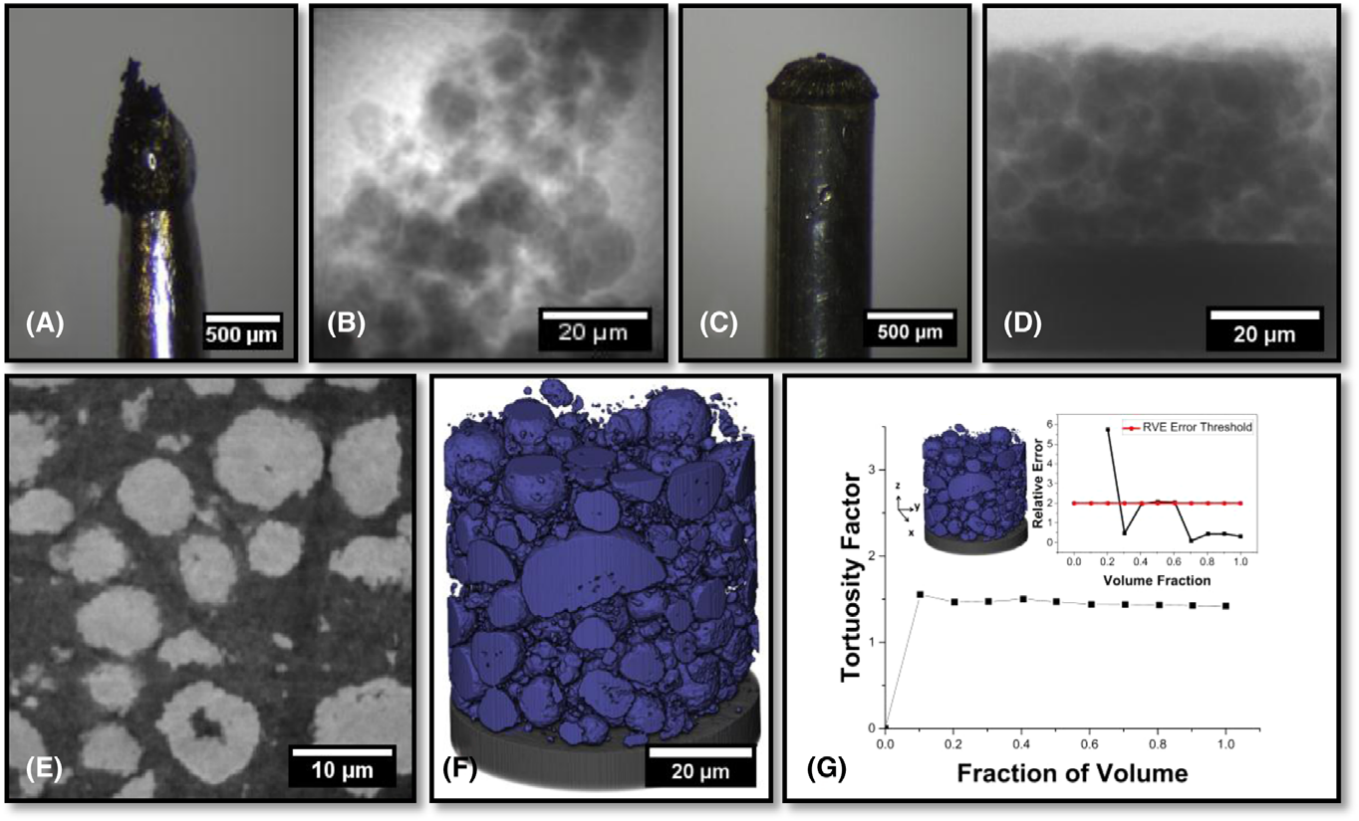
(A) Optical image of traditional mechanical preparation. (B) Corresponding radiograph. (C) Optical image of new laser‐prepared pillar. (D) Corresponding radiograph. (E) Raw orthoslice showing NMC electrode particles. (F) 3D surface rendering of electrode particles (blue) and current collector (black). (G) RVE Analysis of tortuosity calculated in the through‐plane direction, with error inset.
